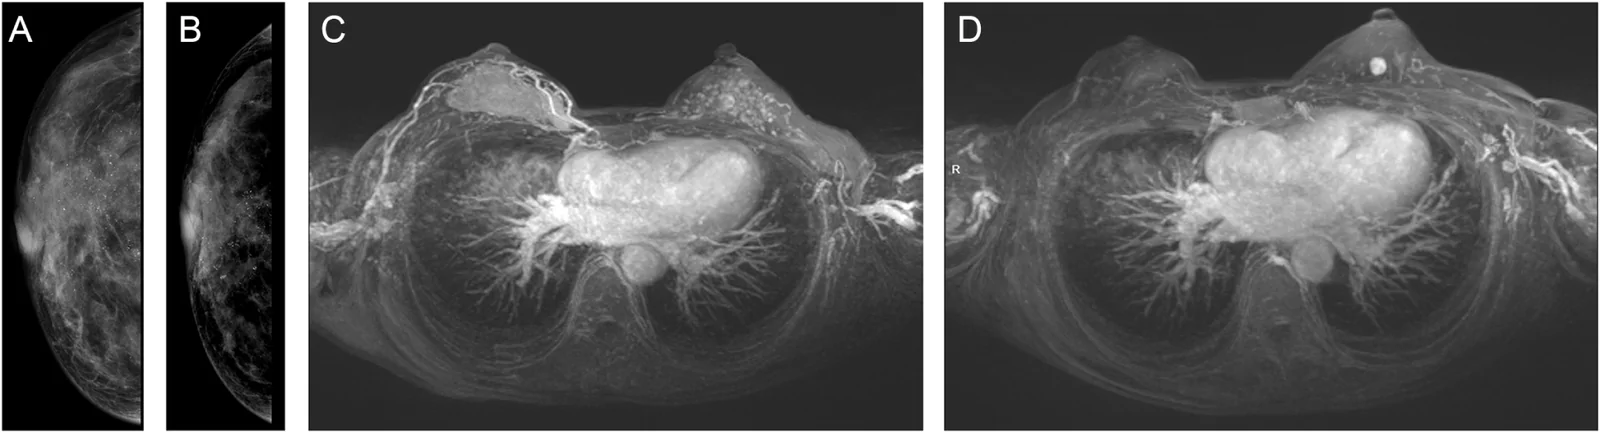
Pre-NAT and post-NAT imaging comparison. (A) Pre-NAT mammogram showing a mass with clustered microcalcifications. (B) Post-NAT mammogram showing a marked reduction in the mass, while calcifications persisted. (C) Pre-NAT breast MRI showing a large, irregular, enhancing lesion in the right breast and enlarged axillary lymph nodes. (D) Post-NAT breast MRI showing near-complete disappearance of the original enhancing lesion, with only a few punctate residual enhancements; the enlarged lymph nodes are no longer visible.
